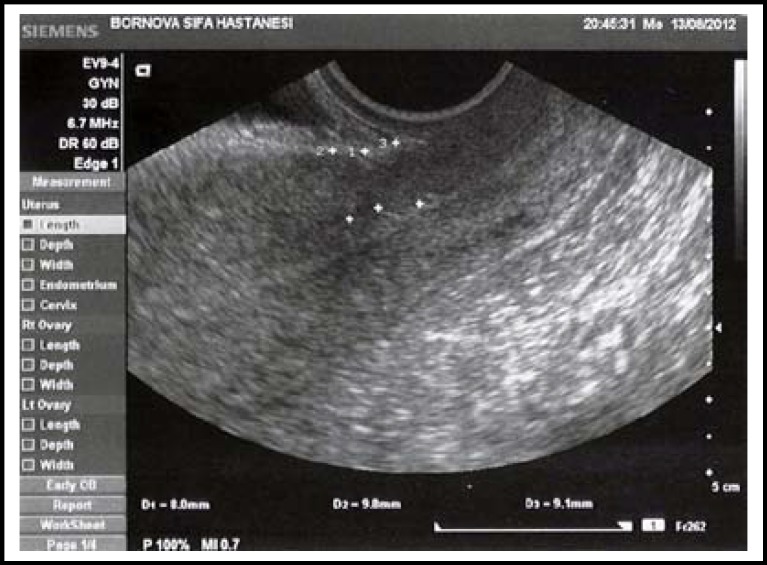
Ultrasound image of a uterus with unlocked method
